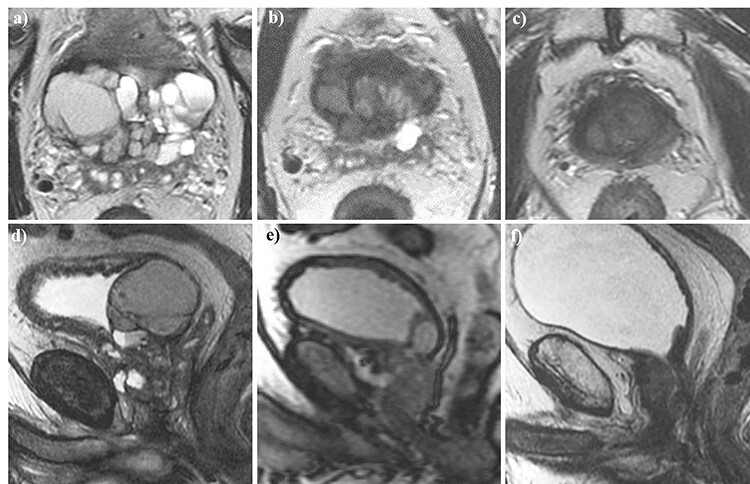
Axial (a–c) and sagittal (d–f) T2W magnetic resonance imaging of the pelvis performed at baseline (a, d), 3 months (b, e), and 3.5 years (c, f) following the commencement of androgen deprivation therapy (ADT). The giant multilocular prostatic cystadenoma observed at baseline showed a significant reduction after 3 months of ADT before resolving completely 3.5 years into treatment.
